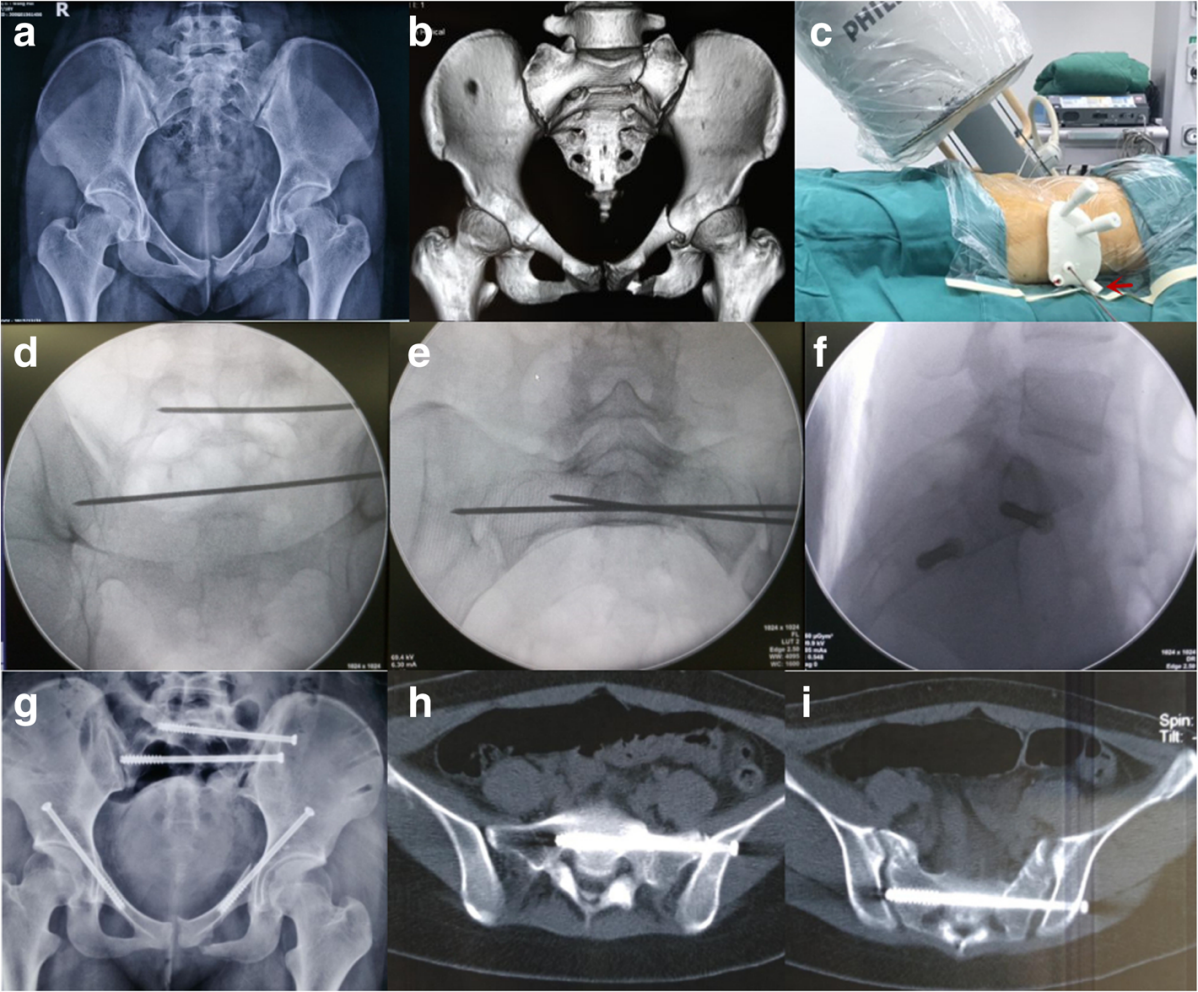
Intraoperative application of the external template. a-b Preoperative AP radiographs/3D reconstruction of computed tomography images for a patient with a Denis zone II sacral fracture and bilateral pubic ramus fractures. c-f Intraoperative fluoroscopy (d/e/f outlet/inlet/lateral view) was used, minimally, to confirm the guide wire and screw in the target corridor. The red arrow in (c) indicates the guide sleeve for the anterior column screw. g-i Postoperative AP radiograph/computed tomography axial image confirmed the placement of the IS screws
